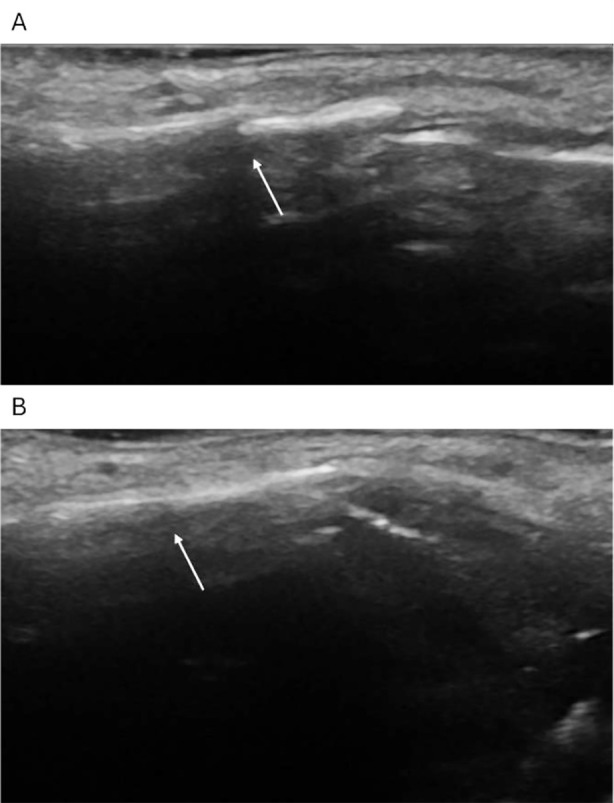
Ultrasound image of a 42-year-old female patient with comminuted nasal bone fracture. A: The hyperechoic zone of bone cortex was interrupted continuously and separated by dislocation. The swelling of soft tissue around the fracture was not obvious. B: After reduction of nasal bone fracture, the fracture ends were well aligned.
